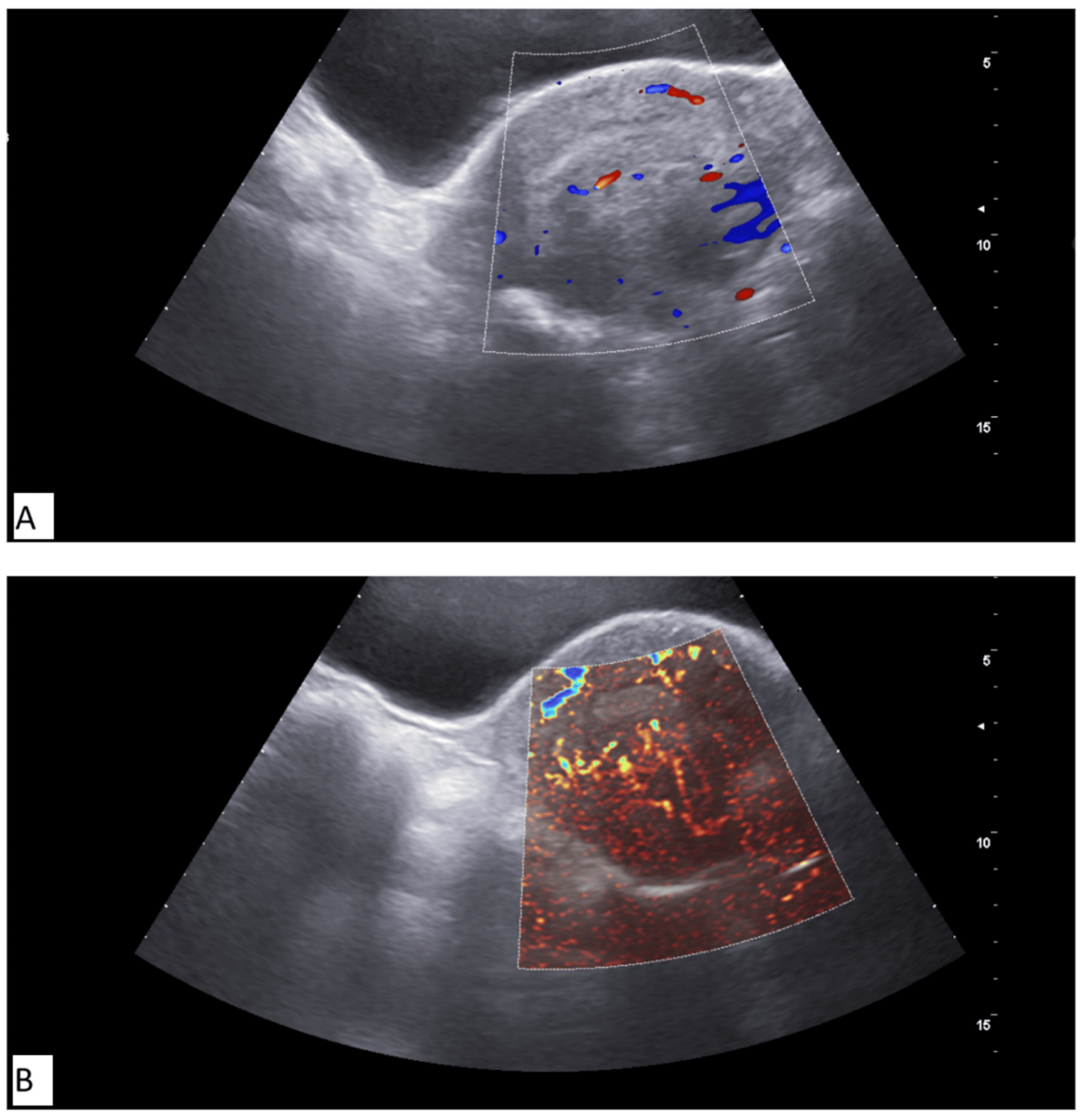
Vascularity of dominant fibroid on color Doppler and SMI (A) Color Doppler showing grade 1 vascularity; (B) SMI showing grade 2 vascularity SMI: superb microvascular imaging
